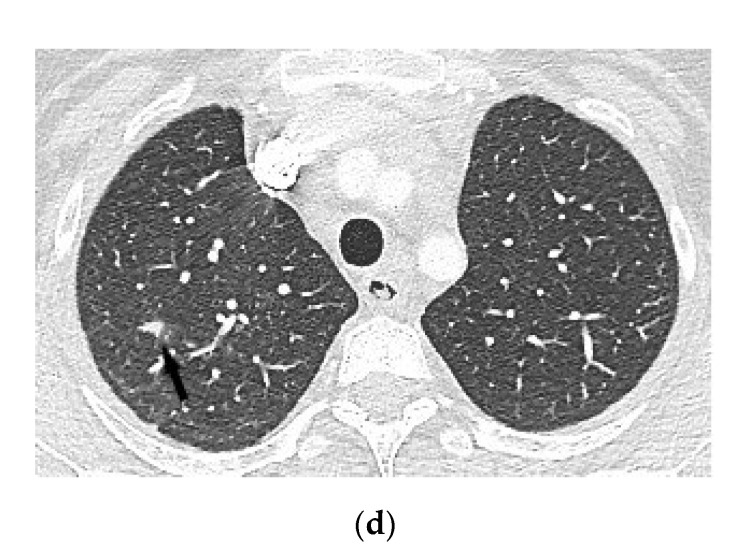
Chest CT scan with contrast enhancement: mediastinal window (a,b) and lung window (c,d) revealed large pericardial effusion up to 4 cm thick (a,b, asterisk), with no significant pericardial thickening, and SVC and IVC dilatation. A small amount of pleural fluid was also demonstrated. Additionally, several small nodules were seen in the apex of the right lung (c,d, arrows).
